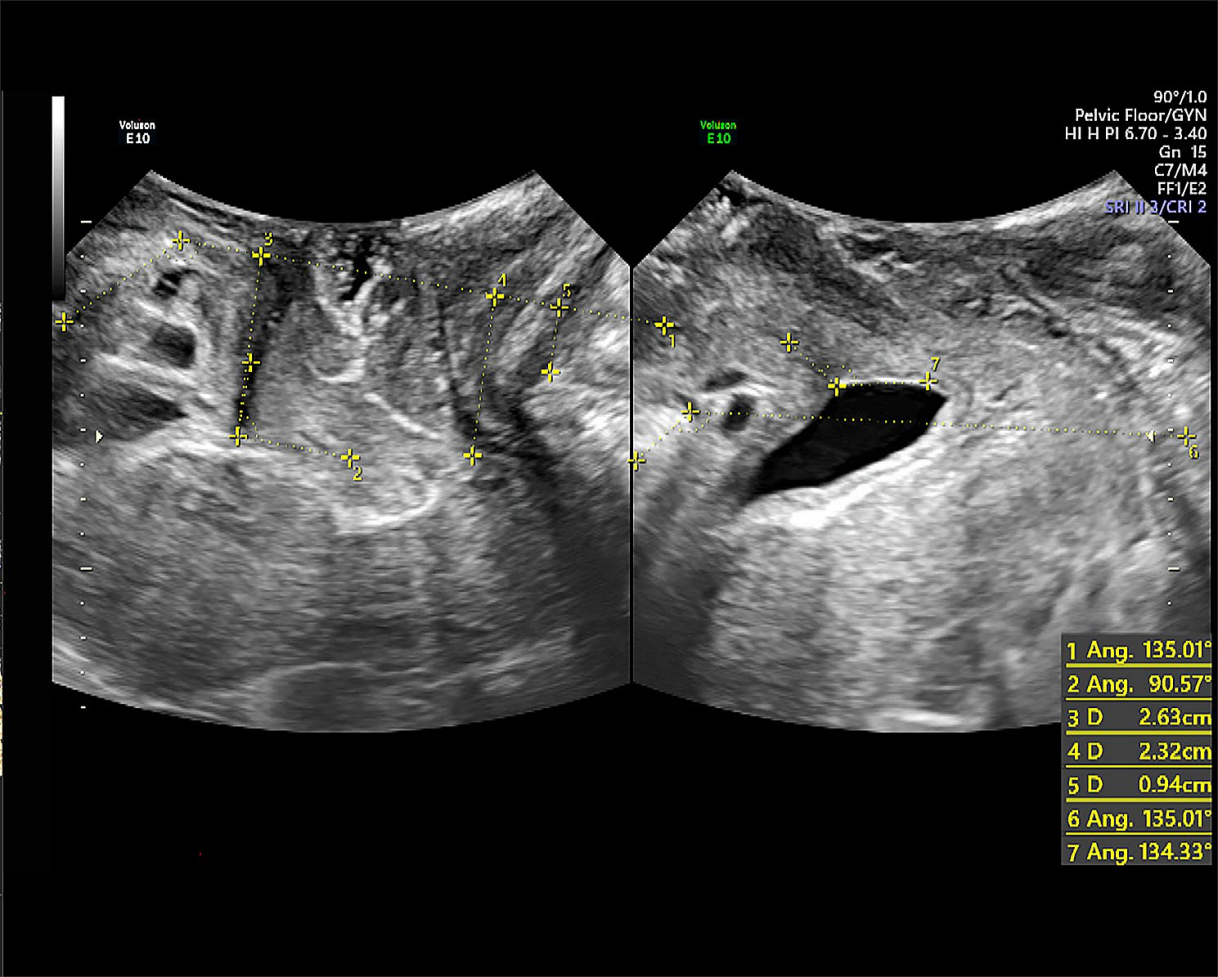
Two-dimensional ultrasound image. (Left) At rest; (Right) At maximum Valsalva. 1 : reference line; 2 : posterior angle of the bladder and urethra; 3 : distance between the cervix and the reference line; 4 : distance between the external cervix and the reference line; 5 : distance between the ampulla of the rectum and the reference line; 6 : reference line; 7 : posterior angle of the bladder and urethra
